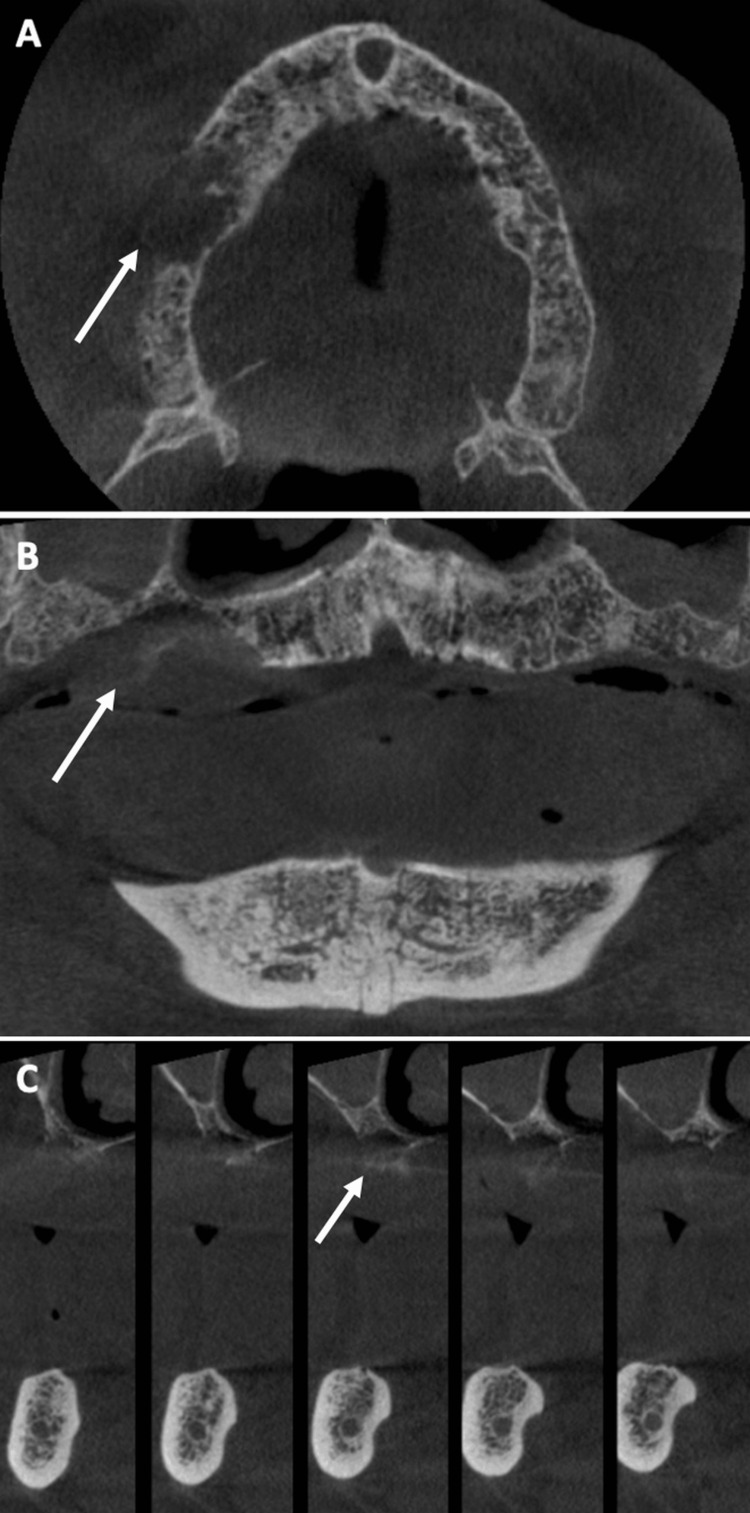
Large osteolysis area could be observed in (A) axial view, (B) frontal view and (C) tangential views of CT scan.
